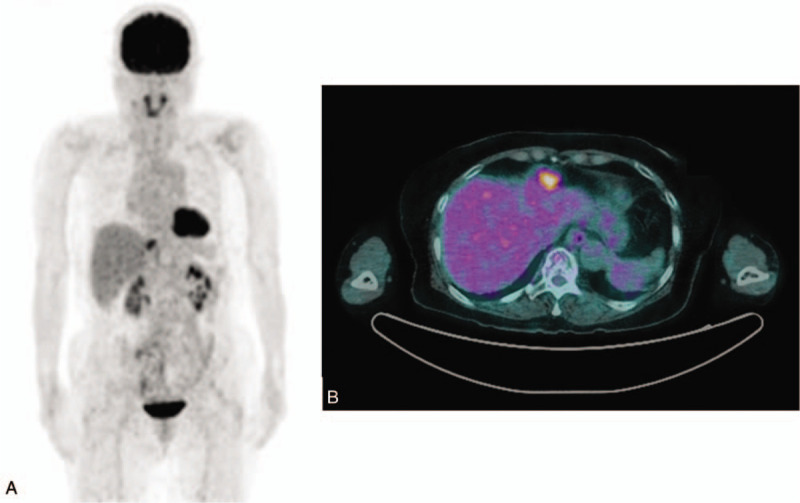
At recurrence (A) and (B) nodular (2.6 cm), markedly intense FDG uptake is apparent in the right sub-diaphragmatic space on positron emission computed tomography/computed tomography.
